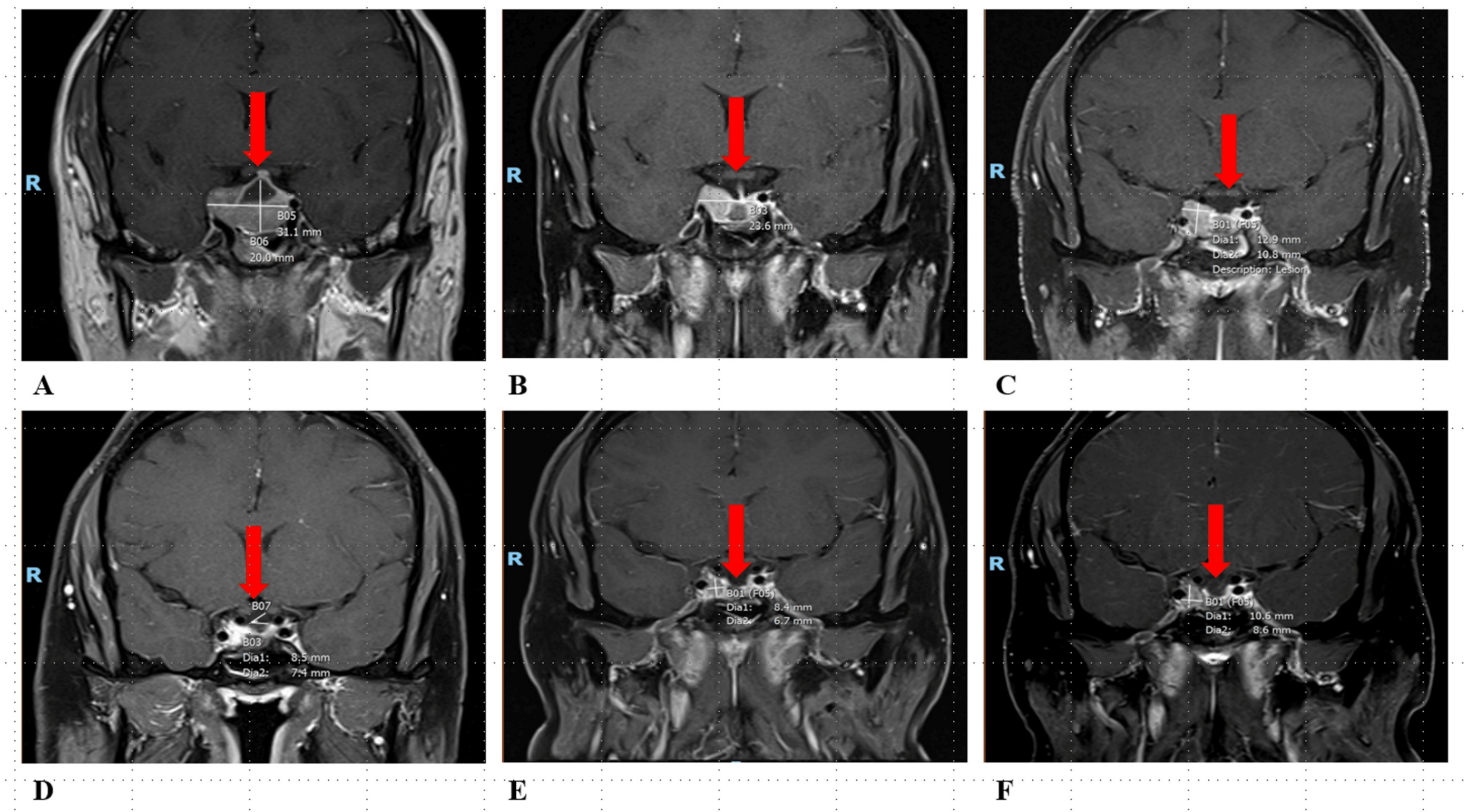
MRI coronal section T 1 post-gadolinium pre- and post-surgical intervention. A: Fall 2018: There is a T1-isointense mixed cystic and solid sellar mass with suprasellar extension. It is slightly increased in size, measuring 1.8x3.0x2.2 cm, with interval-increased cystic component. The mass extends laterally to the right and partially encases the distal right internal carotid artery. There is a mild mass effect on the optic chiasm. B: Mid-summer 2019: Stable enhancing soft tissue mass involving the sella with involvement of the cavernous sinus on the right side. Currently measuring approximately 0.9x1.7x2.4 cm in the craniocaudal, anteroposterior, and mediolateral dimensions. Stable involvement of the cavernous sinus on the right side. There is no mass effect on the optic chiasm. C: Late summer 2019: Postoperative changes of transphenoidal tumor debulking with stable enhancing soft tissue sellar mass. There is a grossly stable appearance of the residual mass measuring 1.1x1.8x2.4 cm with extension into the right cavernous sinus - unchanged mild leftward deviation of the pituitary stalk. D: Fall 2022: Stable appearance of residual pituitary adenoma. The enhancing residual lesion measures 1.9x1.1 cm in the craniocaudal and anteroposterior dimensions. The ventricular system is normal in size and configuration. There is no evidence of mass, mass-effect, hemorrhage, or extra-axial collection. E: Spring 2024: New or increased size of the right sellar/cavernous sinus enhancing lesion. New or increased size of a right sellar/cavernous sinus-enhancing lesion measuring 0.8x0.7 cm. This abuts but does not compress the right cavernous internal carotid artery - no mass effect on the optic chiasm. F: Summer 2024: Postsurgical changes of transsphenoidal pituitary mass resection. Increased size of the right sellar/cavernous sinus-enhancing mass measuring 1.1x0.9 cm in maximal coronal dimensions, with approximately 180-degree encasement of the cavernous ICA without significant vessel compression. No mass effect on the optic chiasm. Red arrow: soft tissue mass.
